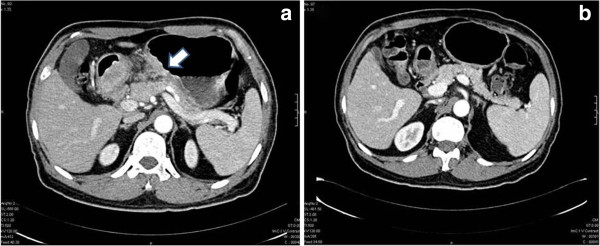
Axial computed tomography scan sections. (a) A patient with gastric cancer demonstrating thickening of the walls of the mid-body along the lesser curvature (short bold, white arrow). (b) Axial section of the same patient demonstrating complete radiological resolution after 3 cycles of NACT. NACT, neoadjuvant chemotherapy.
